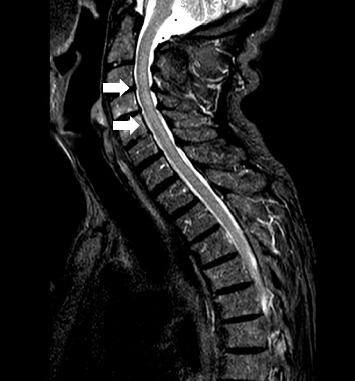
MRI of the spine at 3 weeks (T2 fat-saturated), showing partial resolution of LETM.
